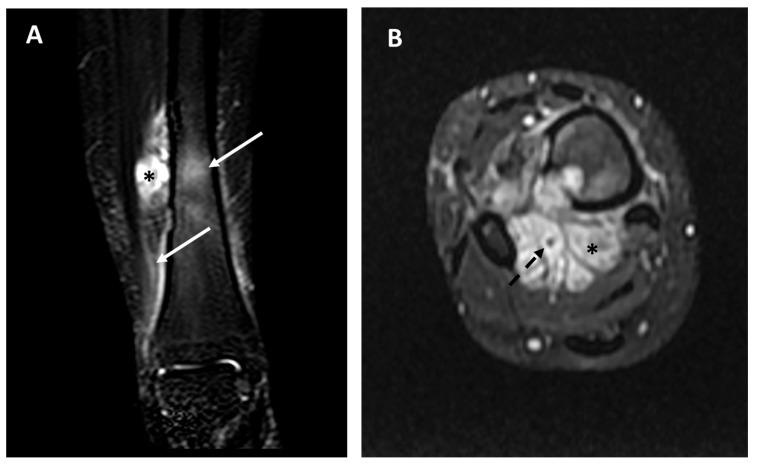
Baseline MRI study in a 26-year-old female affected by adamantinoma of the distal tibia; fluid-sensitive sequences (panel (A), STIR frontal view; panel (B), T2 weighted fat-sat axial view). Extraskeletal components (asterisks), diffuse peritumoral edema (arrows), and vascular encasement (dotted arrow) are well-detectable. This patient developed distant metastases 8 months after the diagnosis and died of disease.
